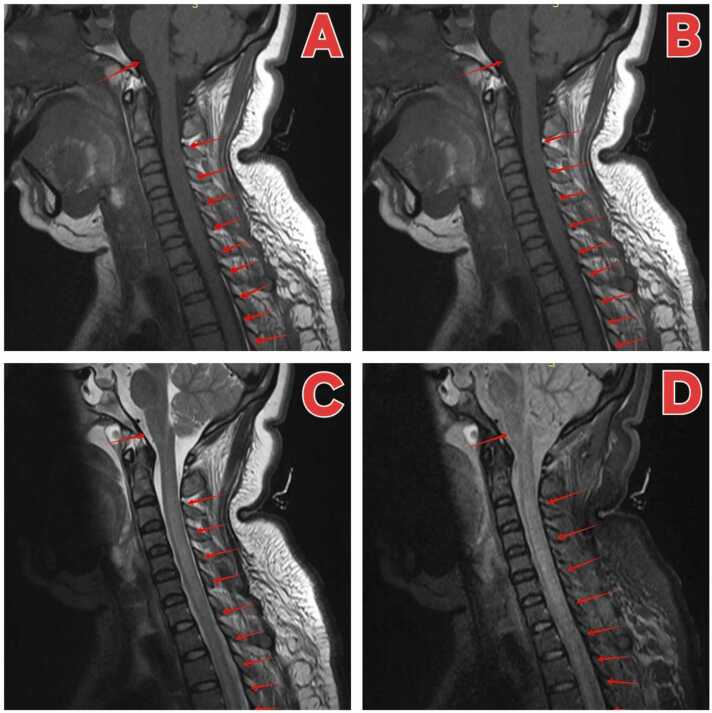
Image(Head and spinal cord MR) findings at the admission. A)sagittal image of plain T2-weighed MRI, B) sagittal image of plain T2-weighed MRI, C) sagittal image of plain T1-weighed MRI,D) sagittal short tl inversion recovery(STIR) MR image.Red arrowhead: original cerebral groove lesion showed no clear indication, linear enhancement of medulla surface, considering infection; abnormal signal of cervical cord and thoracic cord with enhancement, consistent with myelitis.
